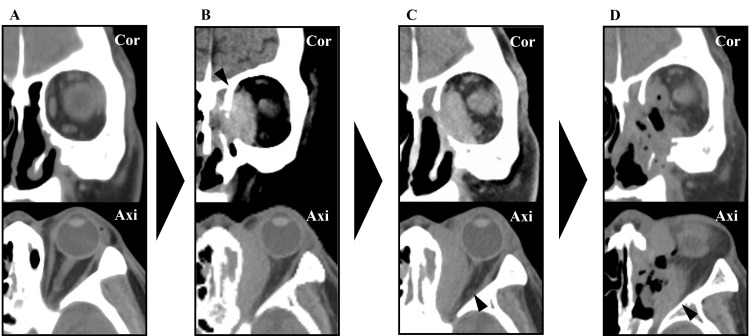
Paranasal Sinus CT Images A: CT scan taken 15 years prior showing an intact medial orbital wall with no evidence of orbital mass; medial rectus and optic nerve appear normal.
